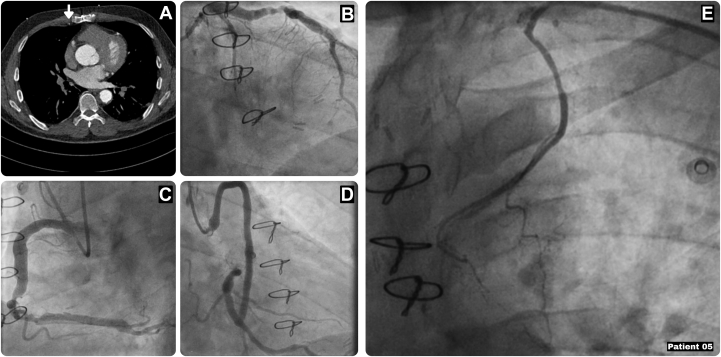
Characteristics of Patient 5 (A) Preoperative computed tomography displaying the RIMA still in situ (arrow). (B) Coronary angiography showing left main disease with severely ectatic native coronary arteries. (C) Coronary angiography showing the ectatic RCA. (D) Coronary angiography showing patent SVG-OM. (E) Coronary angiography showing a nonfunctioning LIMA-LAD graft. LAD = left anterior descending artery; LIMA = left internal mammary artery; OM = obtuse marginal branch; RCA = right coronary artery; RIMA = right internal mammary artery; SVG = saphenous vein graft.
